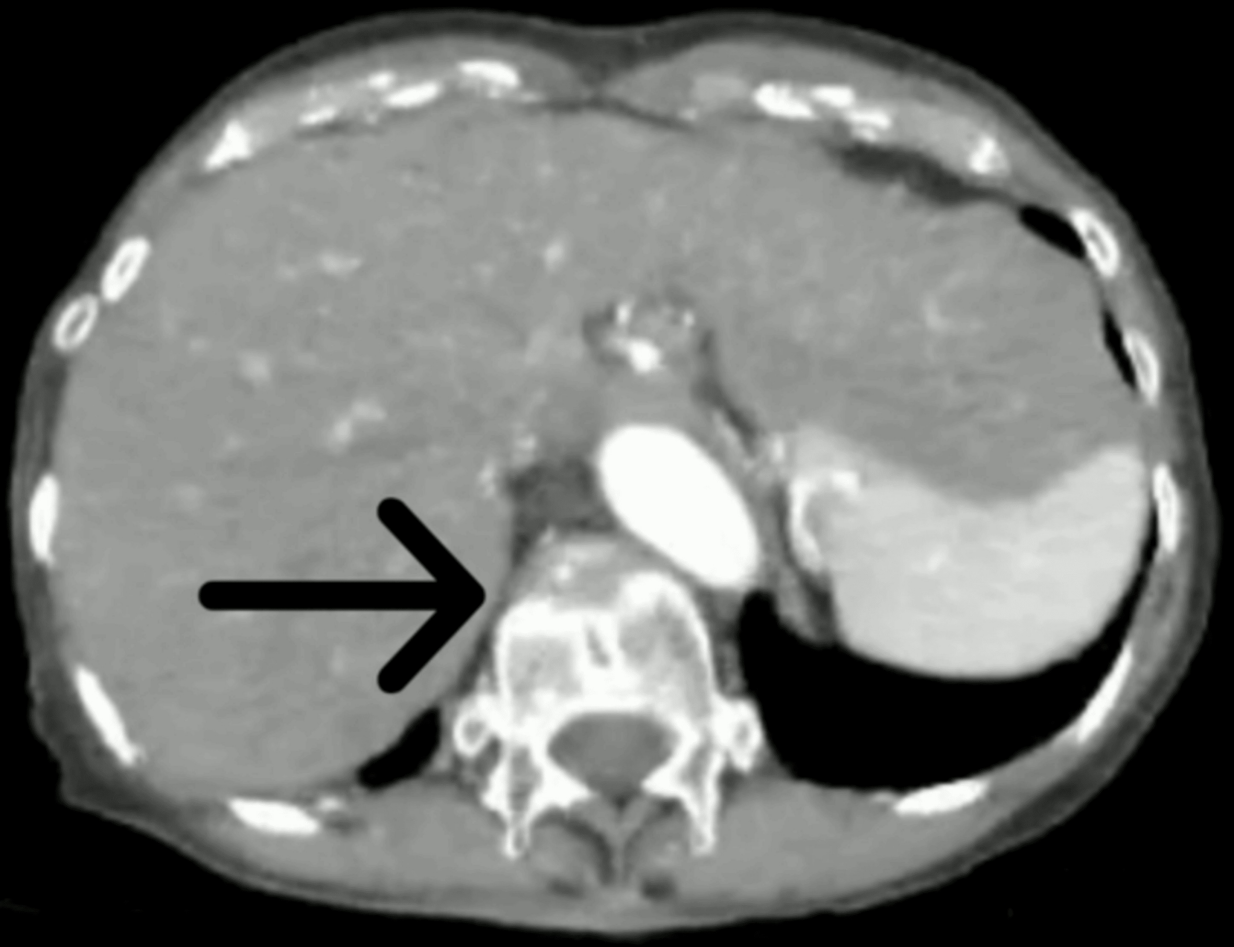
T12 vertebral wedge fracture as indicated by the distortion of vertebral body on axial view CT CT - Computed tomography
